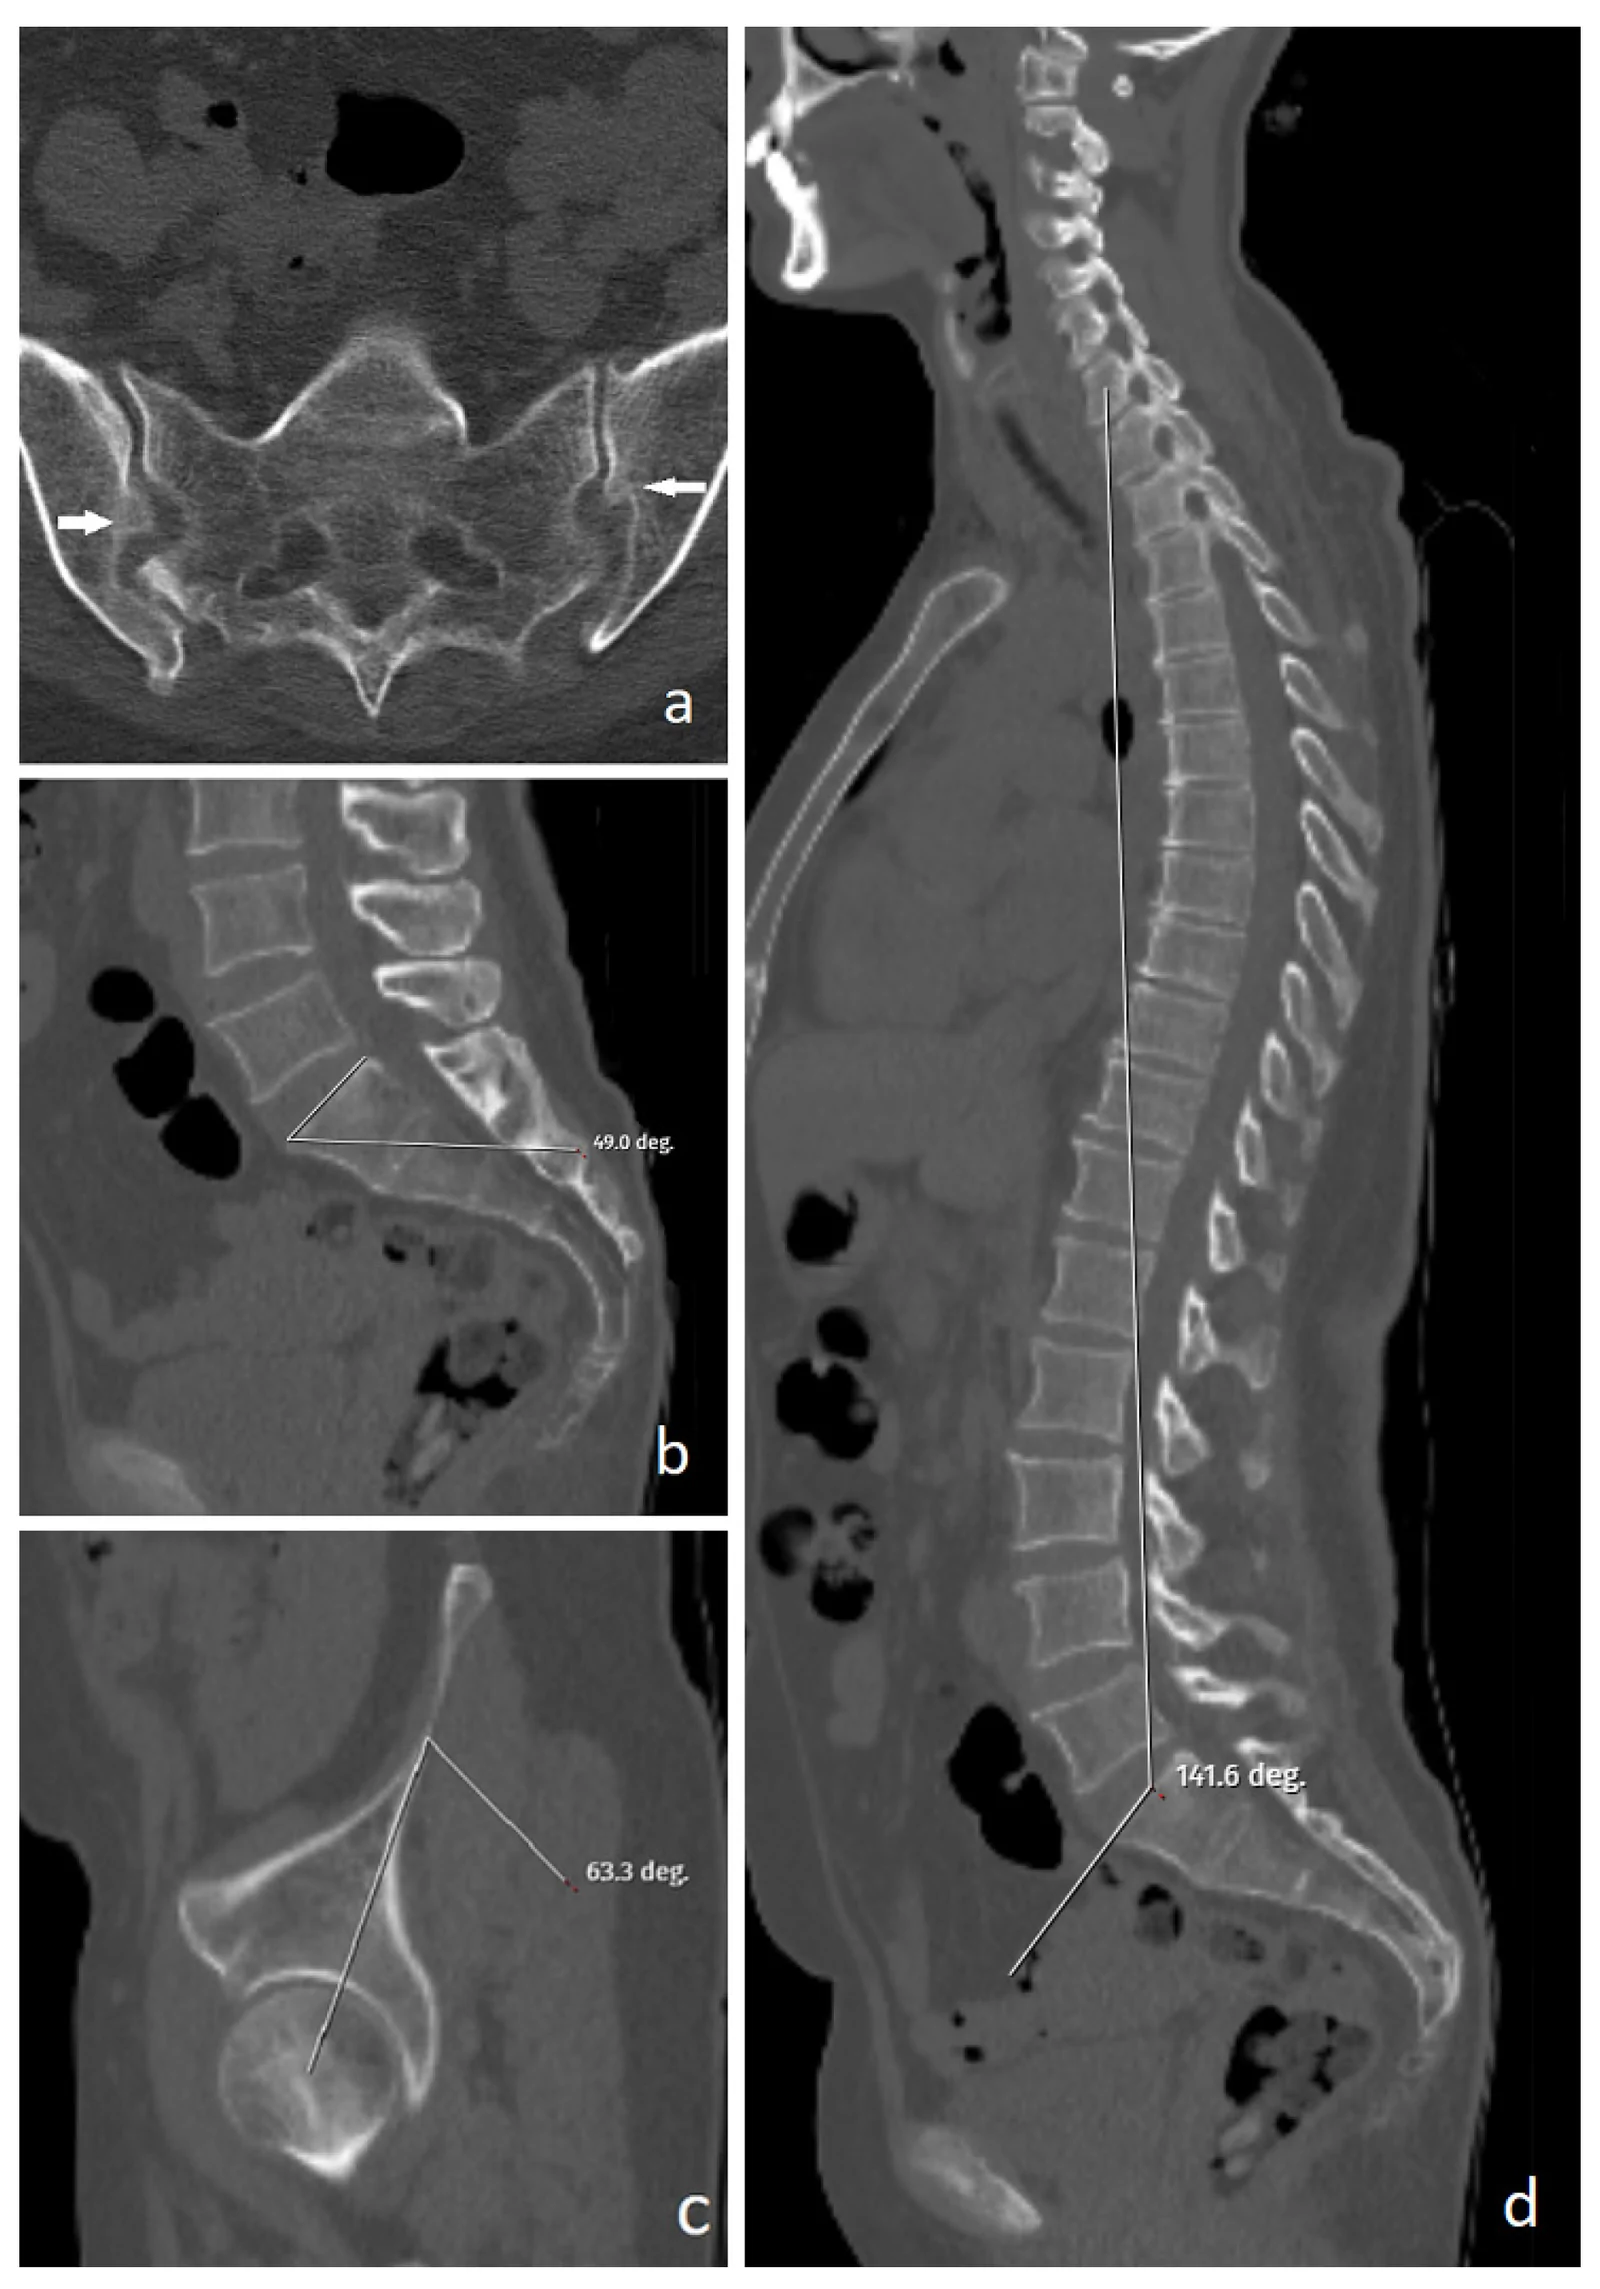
A 67-year-old female who presented to the emergency department with trauma and whose computed tomography imaging revealed no vertebral fracture was found to have iliosacral complex variation in both sacroiliac joints (arrows in subfigure (a)). The patient’s sacral slope, pelvic incidence, and spinosacral angle measurements are given in subfigures (b), (c), and (d), respectively.
